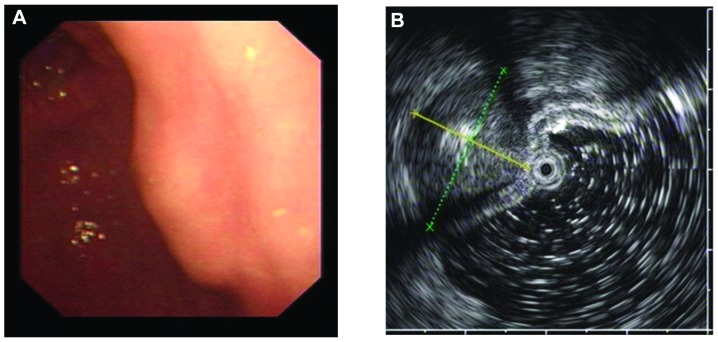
(A) Endoscopic view of the protruding tumor in the gastric antrum. (B) Endoscopic ultrasound image of a 1.5×2.0-cm hypoechoic mass emanating from the muscularis propria with the typical appearance of gastrointestinal stromal tumor.
